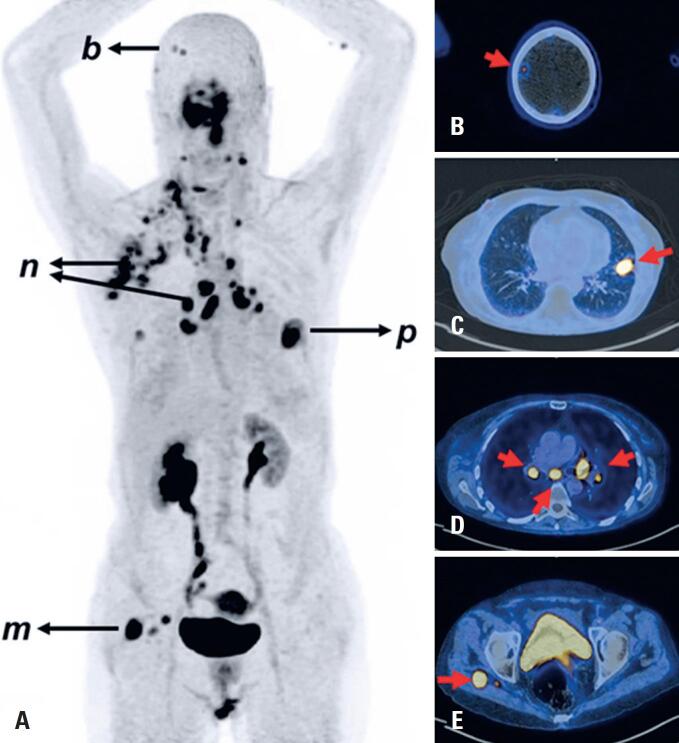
[^68^Ga]Ga-FAPi-46 PET/CT of a 63-year-old woman with non-small cell lung cancer. Whole-body PET/CT data (Biograph mCT scanner, Siemens Medical Solutions, Germany) were acquired 60 min after tracer injection (210 MBq) for 4 min per bed position and reconstructed with an iterative algorithm (A) The maximum intensity projection (anterior view) shows foci of abnormal tracer uptake corresponding with the primary tumor in the left lung (p), metastases in mediastinal, hilar, left supraclavicular, axillary and retropectoral lymph nodes (n), brain (b) and right gluteal muscle (m). (B), (C), (D) and (E) Fused PET/CT axial slices show intense tracer uptake in brain metastasis (B), primary lung tumor (C), mediastinal and hilar lymph node metastases (D), and a metastasis in the right gluteal muscle (E)
